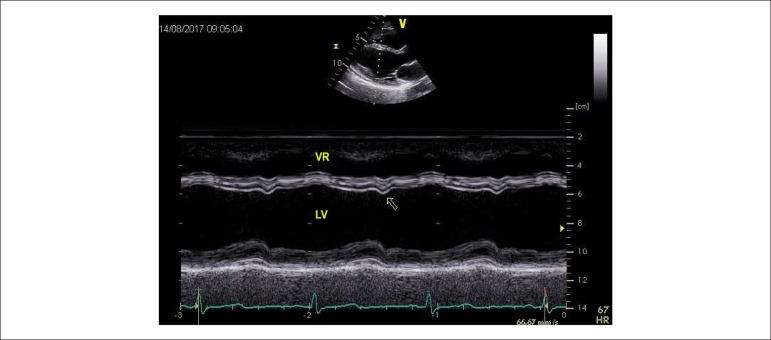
Two-dimensional echocardiography-guided M-mode showing abnormal
